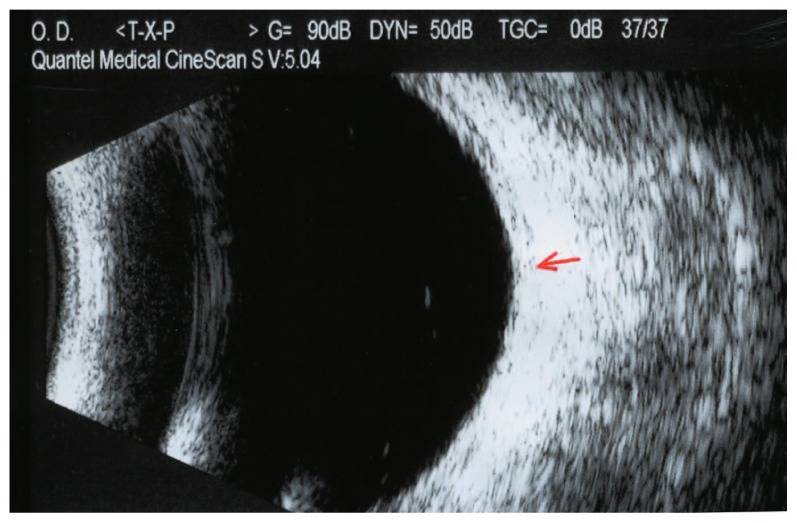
Post-treatment ultrasound scan (January 9, 2008); no mass is detectable and the choroid reveals normal thickness (arrow).
